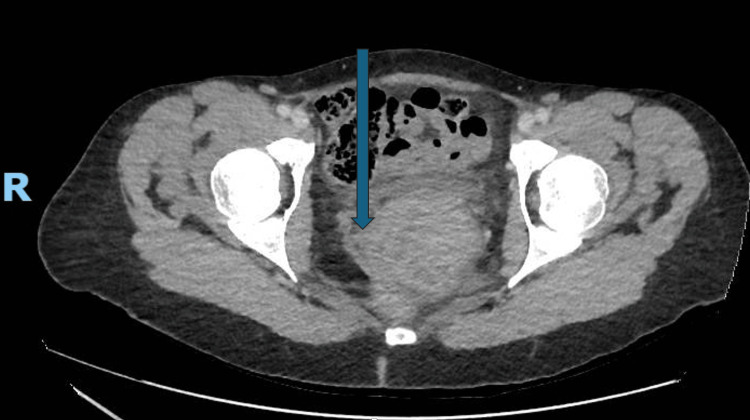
Axial CT pelvis with contrast showing enlarged cervical mass.
